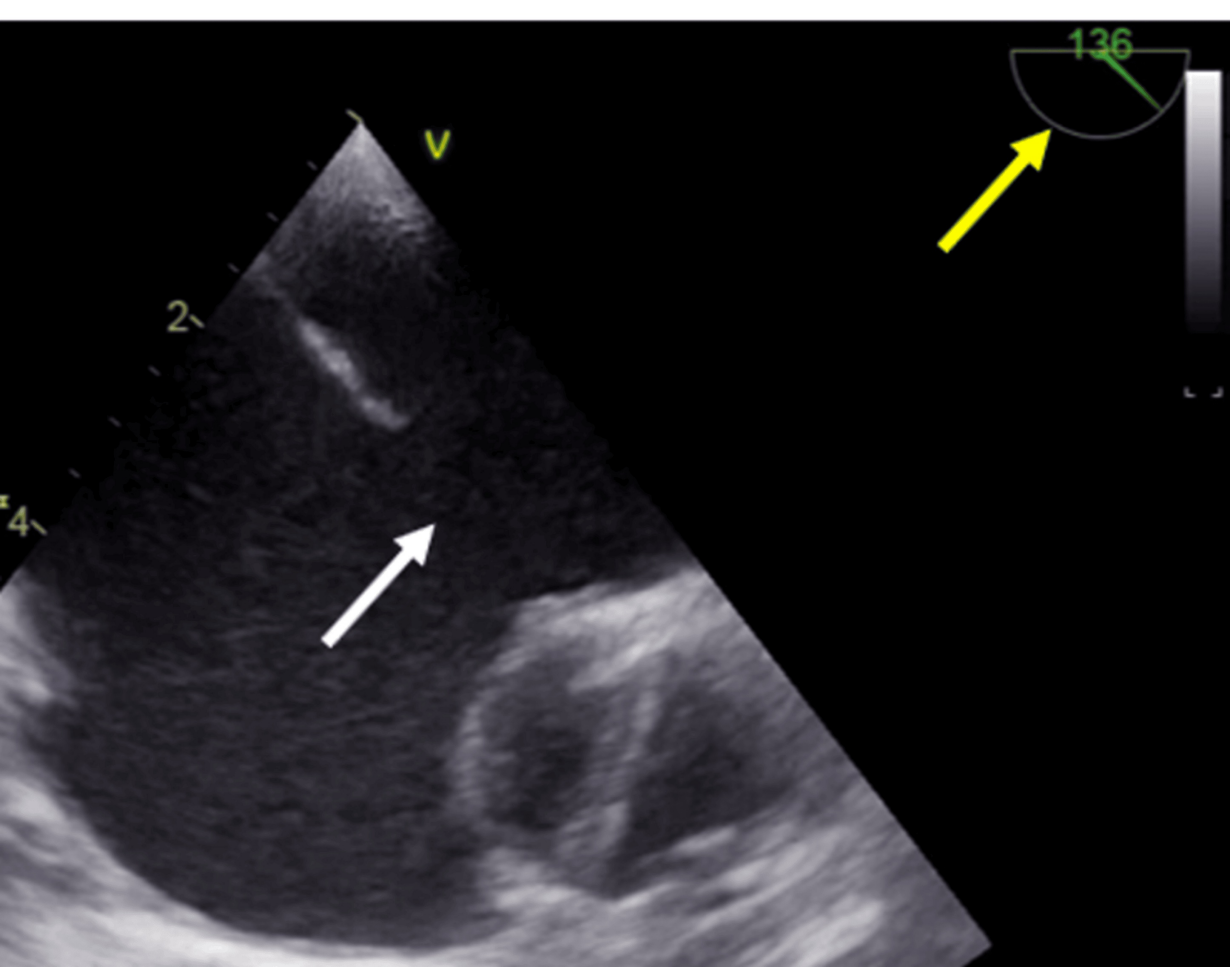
The transesophageal echocardiography (TEE) image obtained at a 130-degree angle mimics the typical appearance of a 60-degree angle due to the altered cardiac orientation. The white arrow indicates the large ASD, and the yellow arrow highlights the TEE imaging angle.
